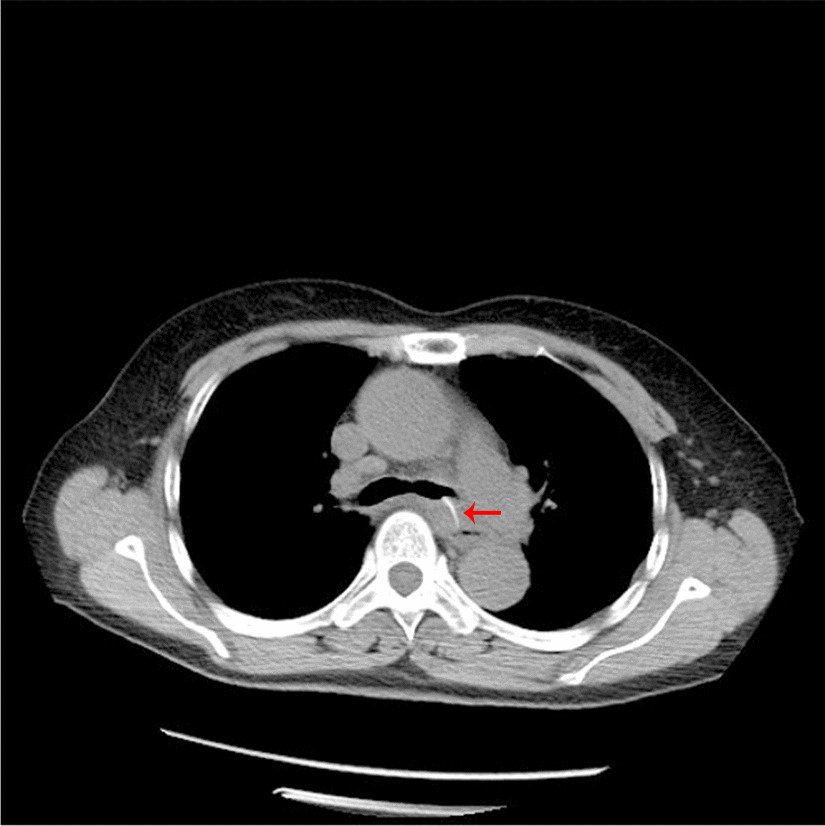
Plain axial CT scan of the chest. The chest CT image shows that a slender fish bone crosses the esophageal wall and considered involves the left main bronchial wall
